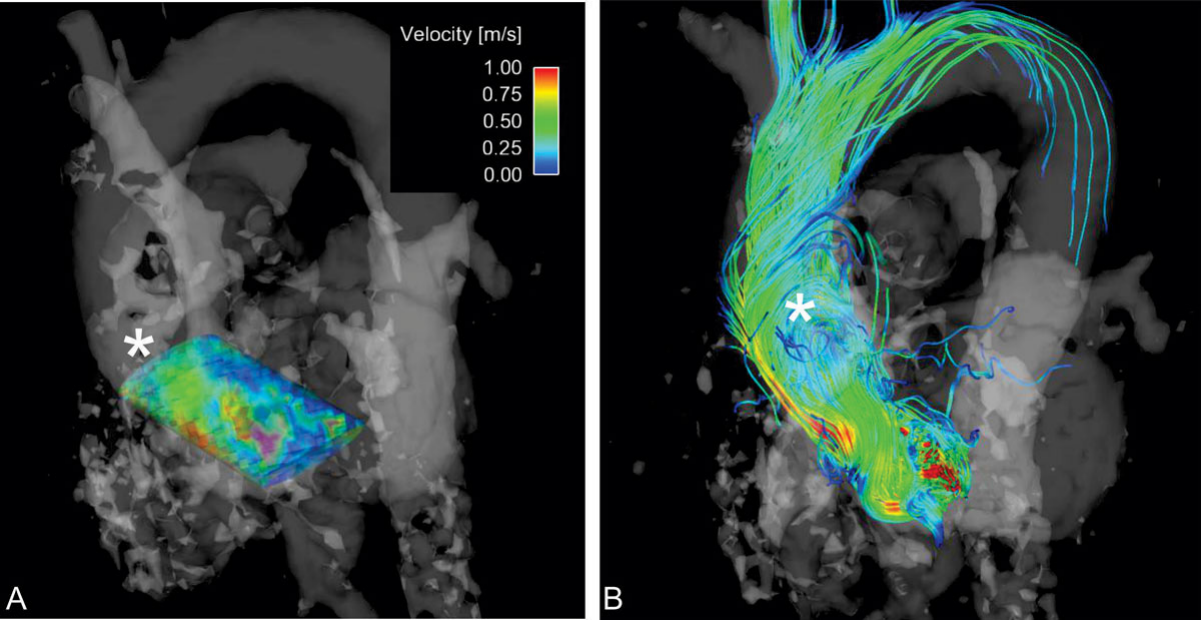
4D flow MRI findings in a patient with obstructive HCM. A) 3D velocity profile in the LVOT at peak systole. This volume was queried throughout the cardiac cycle to capture the peak velocity that was used in the calculation of the MRI-measured outflow tract gradient. B) Streamline representation of flow derangement in the ascending aorta (*). This patient had an average helix grade of 1.5.
